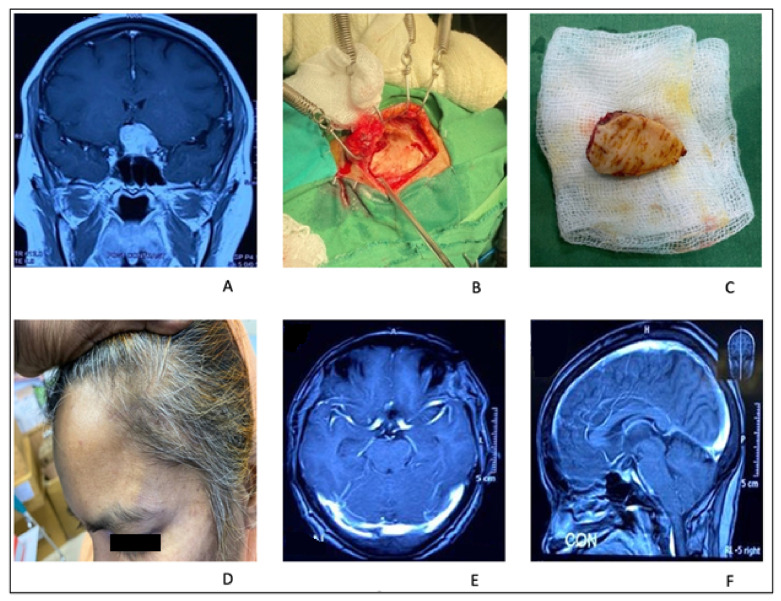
Coronal MRI image of a TSM showing extension of tumor through opticocarotid triangle on left side (A); Mini-pterional craniotomy on left side (B); Small size fronto-temporal craniotomy bone flap (C); Photograph of the patient showing healed scar (D); Post-operative axial and sagittal MRI with contrast showing no residual of the tumor (E,F).
